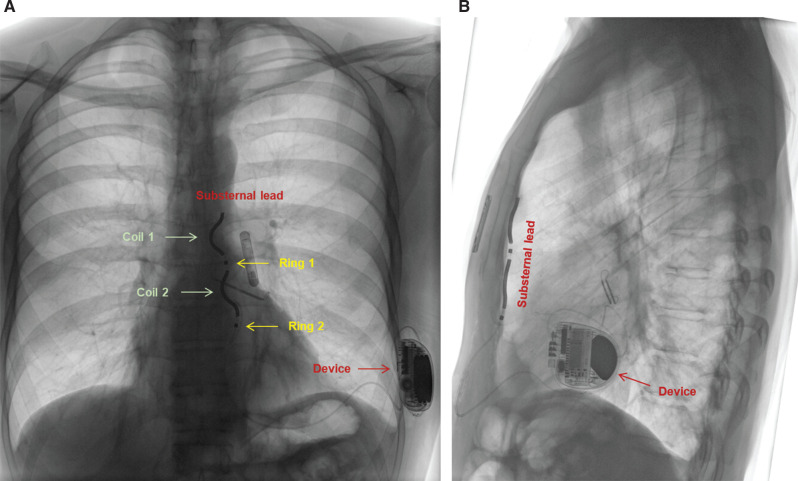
A chest X-ray taken after extravascular implantable cardioverter-defibrillator implantation showing the correct position of the lead substernally (B) and of the coils facing toward the patient’s right side, while the sensing electrodes (Ring 1/Ring 2) are facing toward the left (A).
